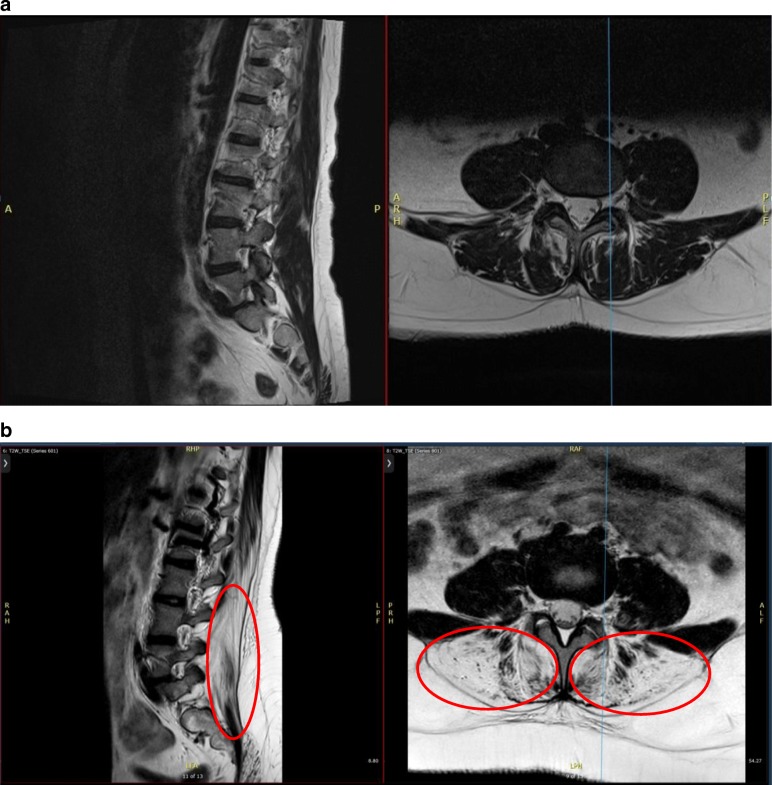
a Normal MRI. b Case report MRI images, sagittal and axial T2-weighted slices, from the lumbar spine demonstrate paraspinal muscle atrophy with fatty tissue replacing muscle, evident as high T2 signal, in the patient (circled)
